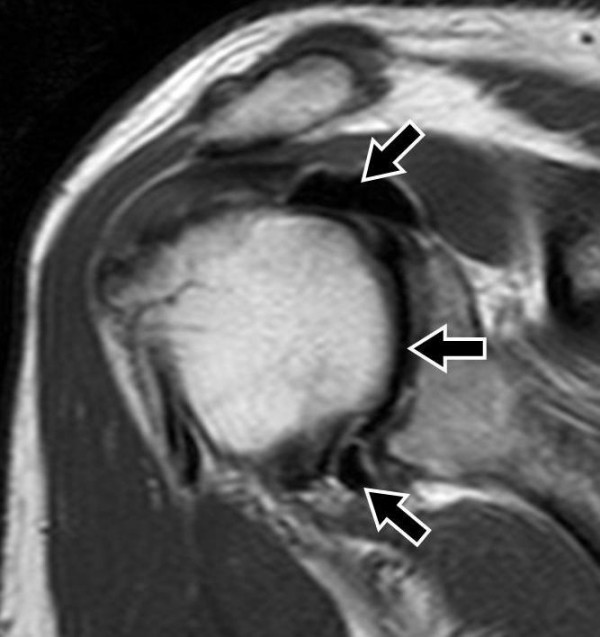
SE T1-weighted sequence (TR/TE/Thick: 500 ms/10 ms/4 mm) on oblique coronal plane after administration of dimeglumine salt of gadopentetate acid diluted in saline solution at 0.5 mol/l concentration. Artefact is detectable, characterized by contrast signal absence (black arrows), with no evidence of contrast agent in peri-articular soft tissues.
